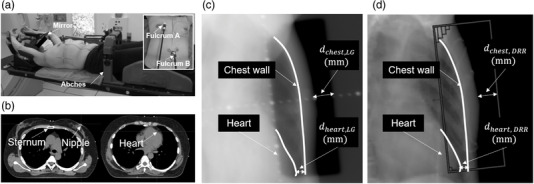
(a) The typical setup of Abches for a patient with left‐sided breast cancer. Fulcrum A is placed on the patient's abdomen and Fulcrum B is placed on the contralateral chest to measure the deep inspiration breath‐hold (DIBH) signal; (b) measurement points for the sternum, nipple, and heart; (c) a linac graph (LG) acquired before the treatment; (d) a digitally reconstructed radiograph (DRR) reconstructed from treatment planning CT image. Edge lines of the heart and chest wall are defined as white lines in each image
